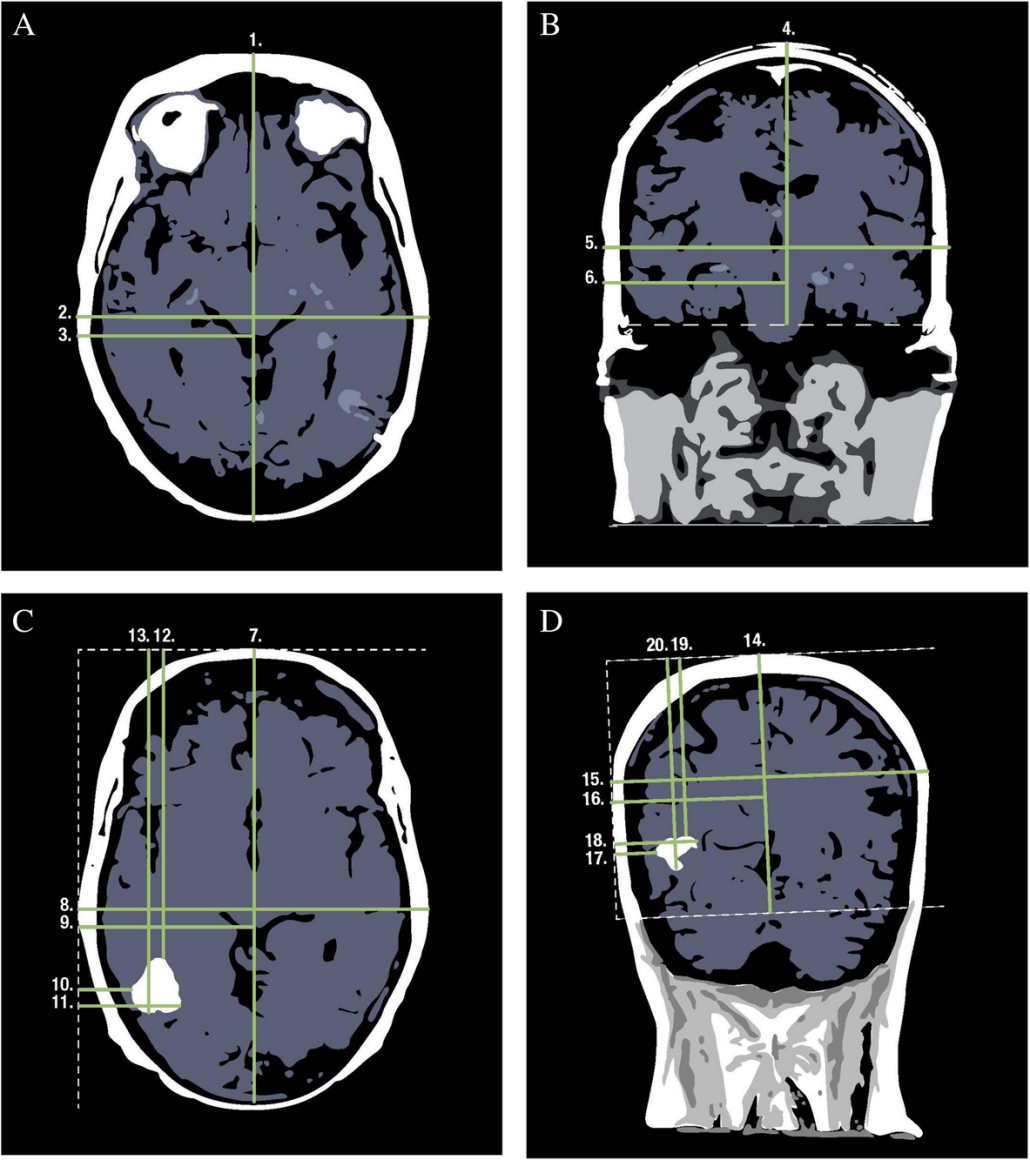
Magnetic resonance imaging. a Axial T1 sequence slice containing most apical image, including orbits used to measure head size. b Coronal T1 sequence slice selecting image at level of ear canal used to measure head size. c Postcontrast T1 axial image shows maximal enhancing tumor diameter used to measure tumor location. d Postcontrast T1 coronal image shows maximal enhancing tumor diameter used to measure tumor location
